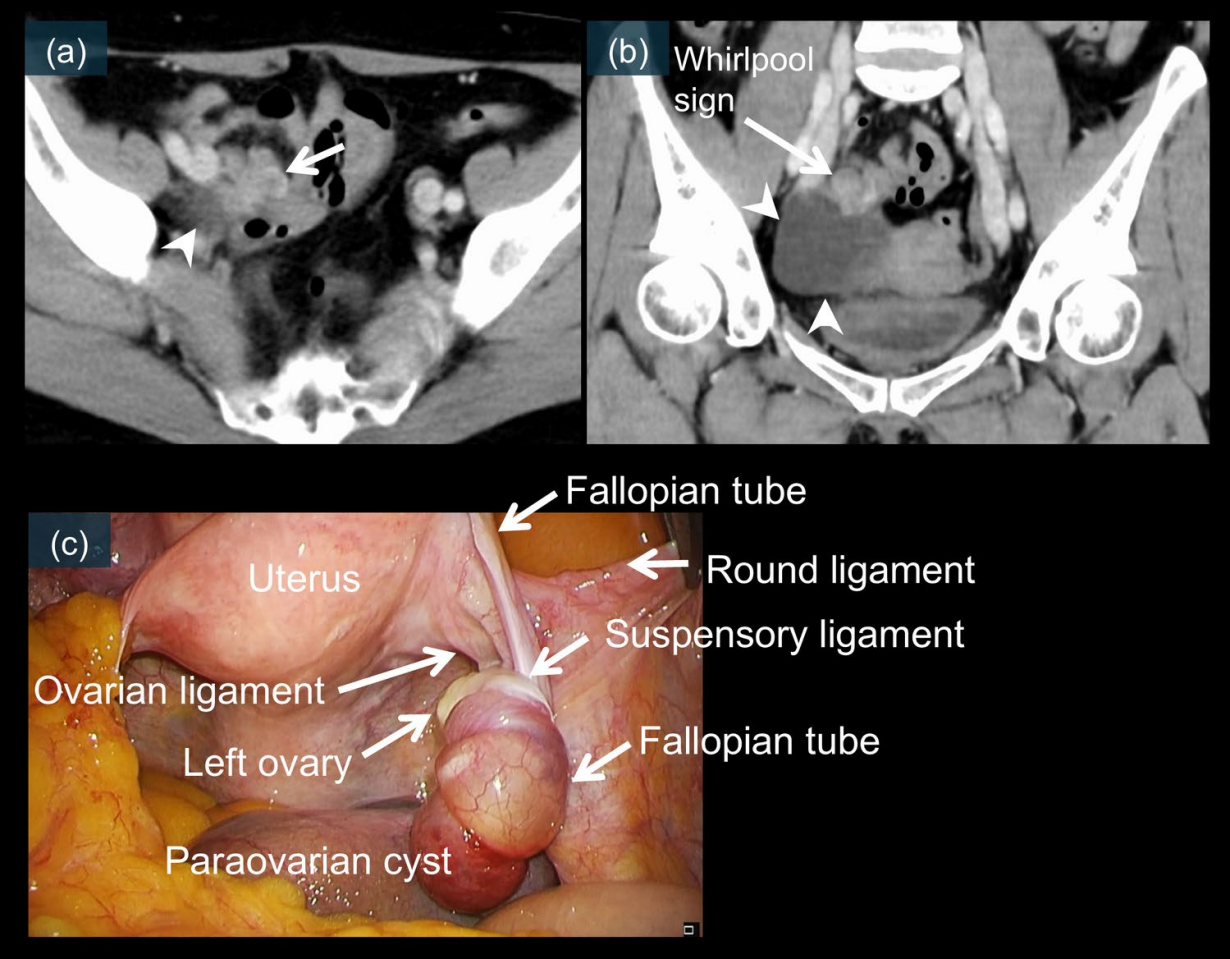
A 60-year-old female. Contrast-enhanced CT images (a and b) show a 4.4-cm mass in the pelvis (white arrowheads). Adjacent to the mass, a whirlpool sign is observed (b), which is considered to be a twisted pedicle (a and b, white arrows). (c) is a laparoscopic image, which reveals a 720° torsion of the right paraovarian cyst
